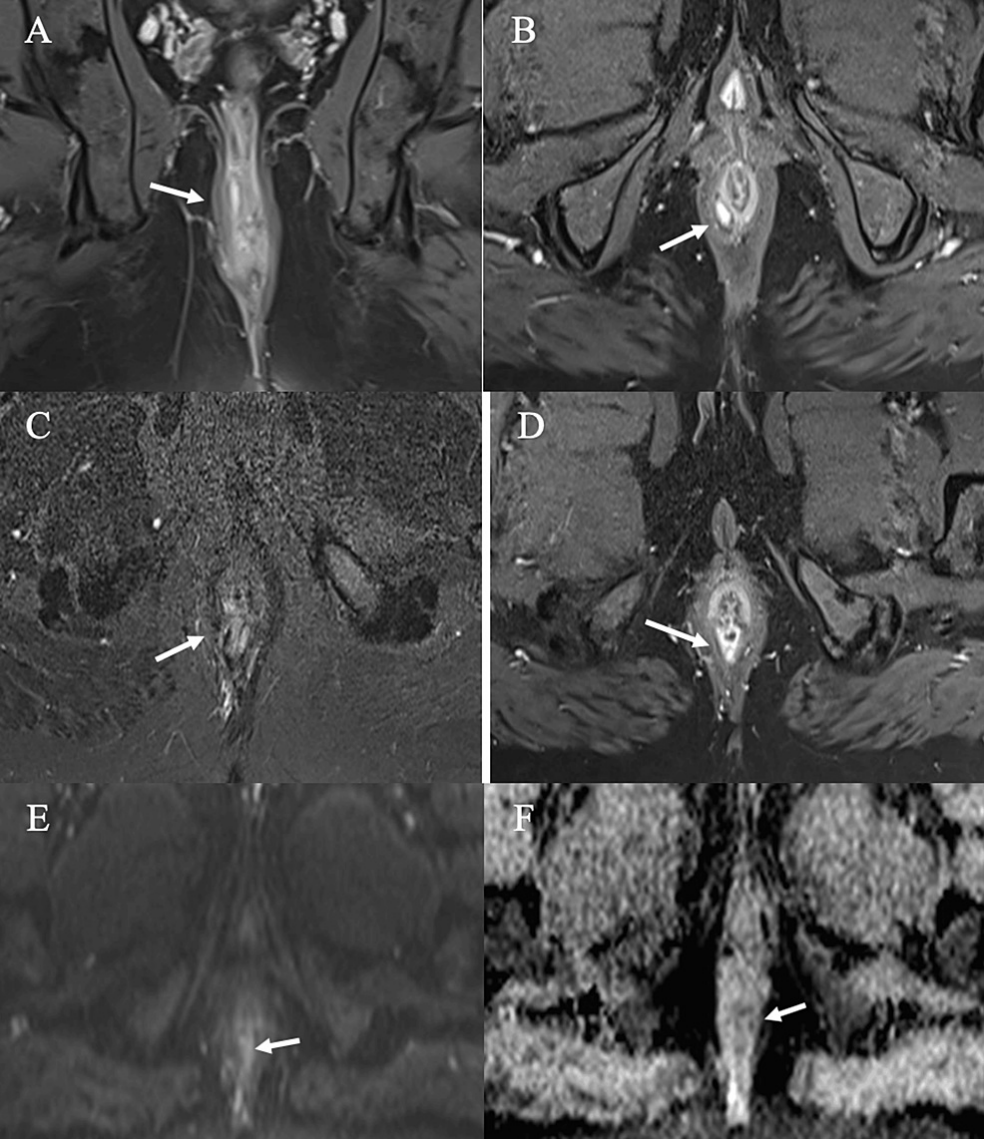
Grade 2 intersphincteric fistula with abscess. (A-B) Coronal STIR and axial post-contrast fat-suppressed T1-WI showing an intersphincteric fistula. (C-F) Axial STIR, post-contrast fat-suppressed T1, DWI, and ADC, respectively, depicting mild collection showing a hyperintense signal on T2 STIR (C), peripheral rim enhancement on the post-contrast image (D), and restricted diffusion DWI (E) with a drop on ADC map (F). DWI: diffusion-weighted imaging, STIR: short tau inversion recovery; ADC: apparent diffusion coefficient
